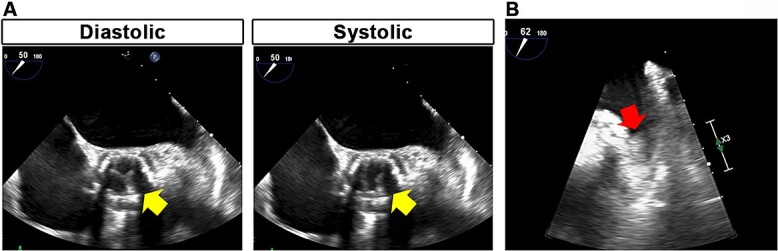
Transesophageal echocardiographic images. Reduced leaflet motion of the bioprosthetic valve (A, arrows) and left atrial appendage thrombus (B, arrow) were detected.
